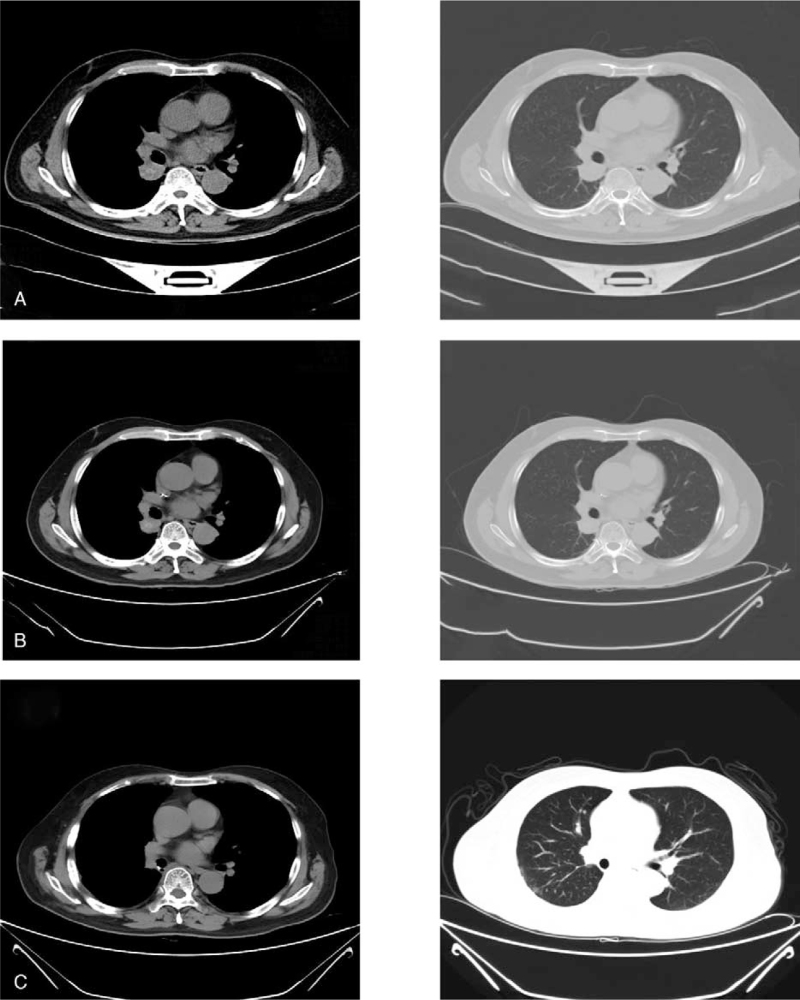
Data after neoadjuvant therapy 06-24-2021 (A): after 2 courses of neoadjuvant therapy, compared with the chest: the tumor in the dorsal segment of the lower lobe of the right lung was significantly smaller that of the CT imagery from 4-22-2021; the area was about 23 mm × 29 mm, the edge was smooth, and the surrounding exudate had been absorbed. The nodule in the middle lobe of the right lung was the same as before. The mediastinal and right hilar lymph nodes were smaller than before. 07-03-2021 (B): Before the third course of neoadjuvant therapy, the mass continued to shrink; the area was about 18 mm × 26 mm. 02-18-2022 (C): To date, we have observed no recurrence or obvious adverse reactions.
